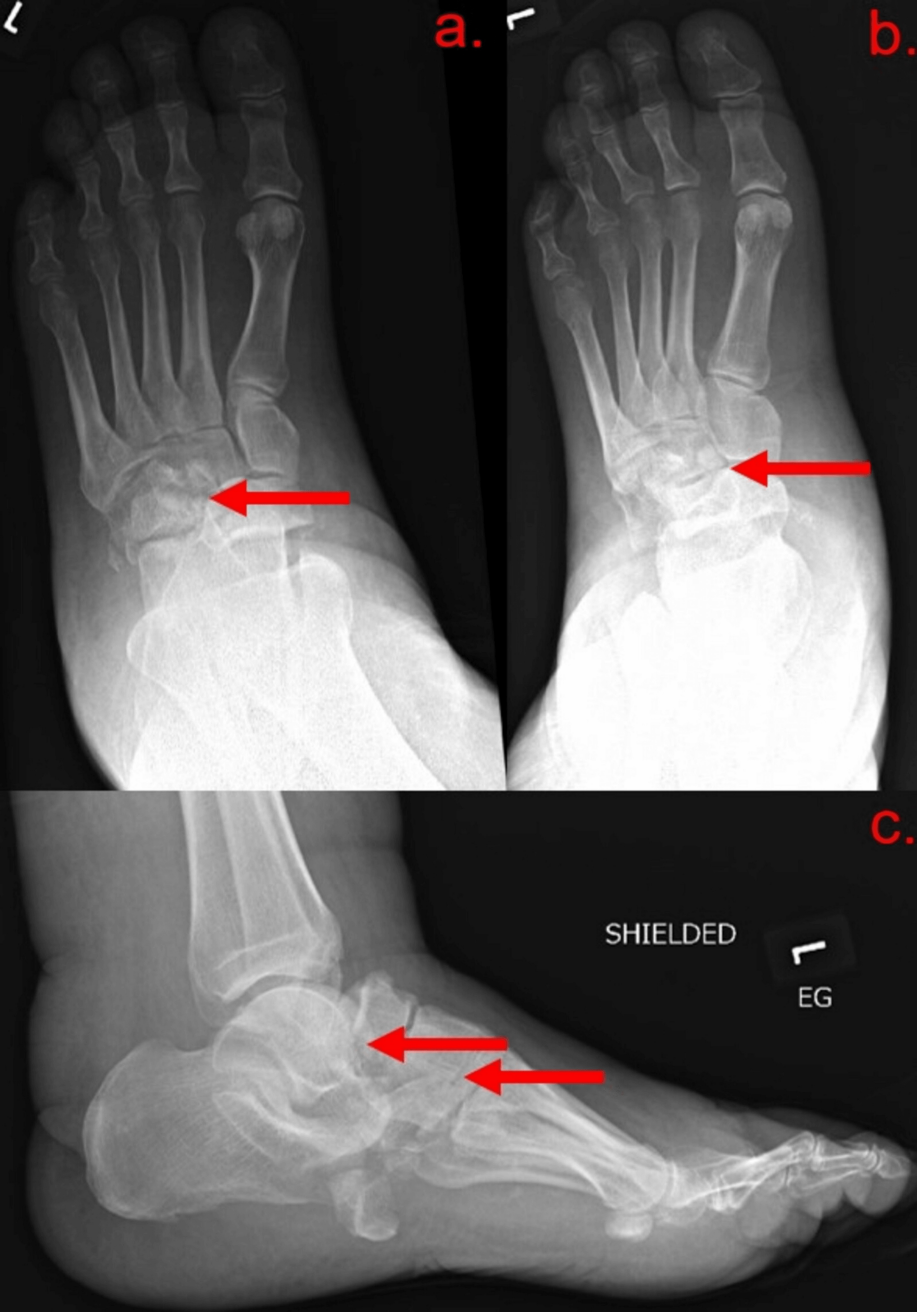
X-ray of the left foot in (a) anteroposterior (AP), (b) oblique, and (c) lateral views X-ray of the left foot in (a) AP, (b) oblique, and (c) lateral views, demonstrating extensive acute comminuted displaced intra-articular fractures of the left hindfoot with involvement of the talus, cuboid, navicular, and likely lateral cuneiform (red arrows). There is dislocation of the talocalcaneal and talonavicular joints. The left cuboid is significantly displaced. There is severe soft tissue edema.
